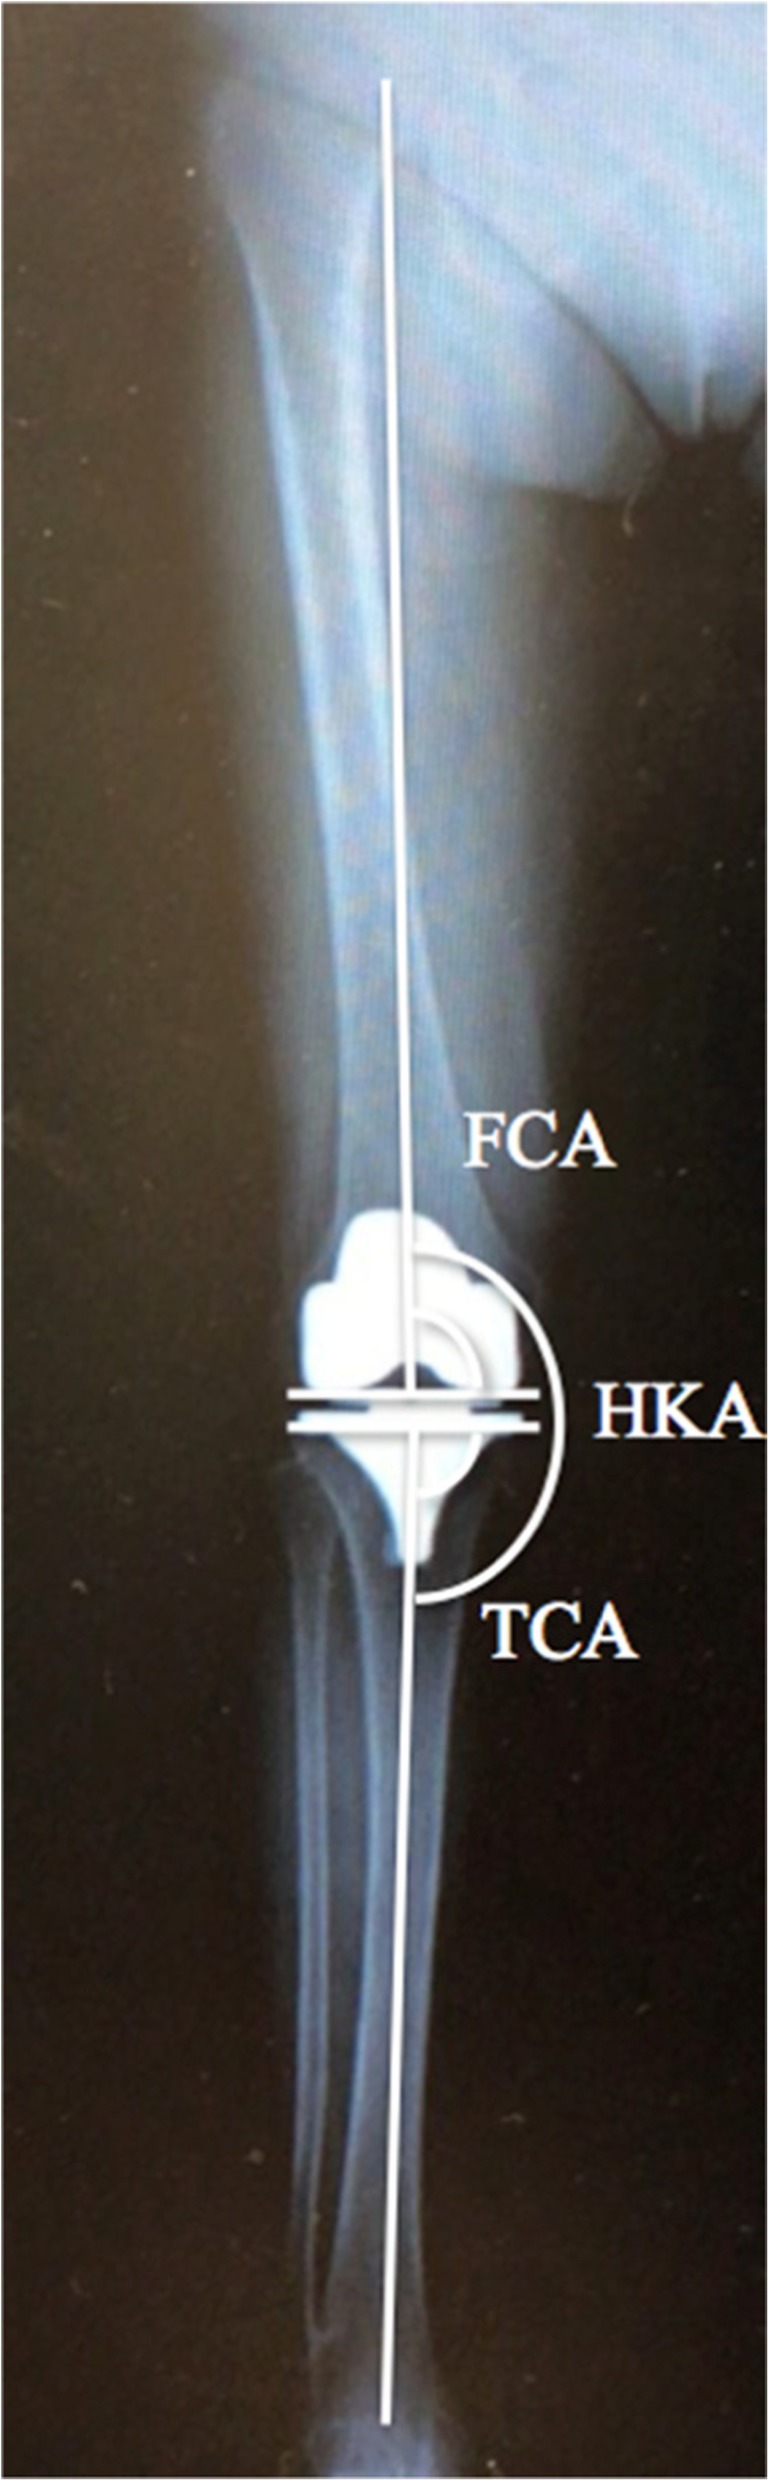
Radiograph of the postoperative component alignment in the coronal plane. HKA was measured as the *white line*. FTA was measured as the angle between the femoral shaft axis and tibial shaft axis. *FCA* femoral coronal angle, *FTA* femoral–tibial angle, *HKA* hip–knee–ankle angle, *TCA* tibial coronal angle
